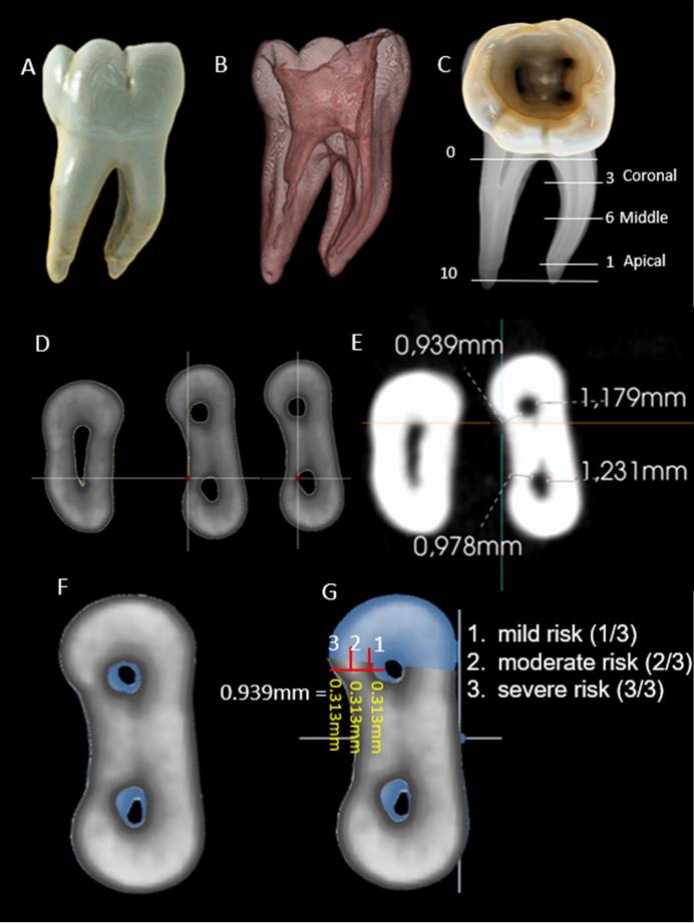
CBCT scans show the volumetric reconstruction and the regions selected to be evaluated (A-E). The measurements were taken along the root canal (coronal, middle, and apical thirds) of the lower cementum-dentin thickness (i.e., coronal third - 3 mm from the furcation; medium third - 6 mm from the furcation; apical third - 1 mm short from the apical foramen)(C-E). Measurement of cementum/dentin thickness before (axial slice in blue) and after (axial slice in gray) RCP in all root thirds, distributed into three (3/3)
